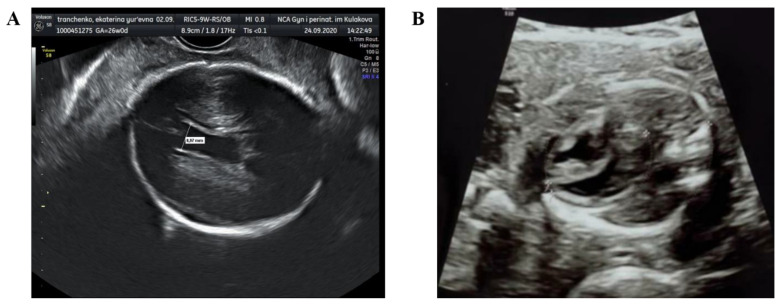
Ultrasound fetal heart scans at the 25th week of gestation: (A) intraventricular hemorrhage; (B) myocardial hypertrophy.
